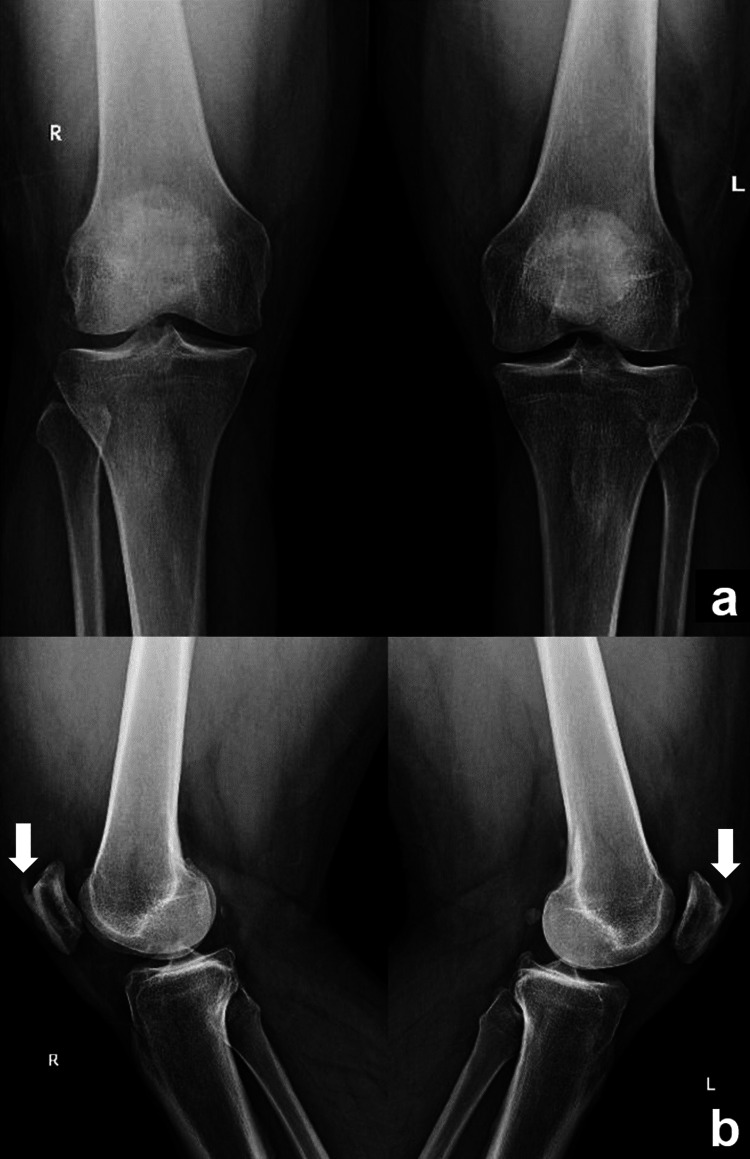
Bilateral knee anteroposterior (a) and lateral (b) radiographic views. White arrows indicate the bony spurs (enthesophytes) at the superior poles of the patellae
